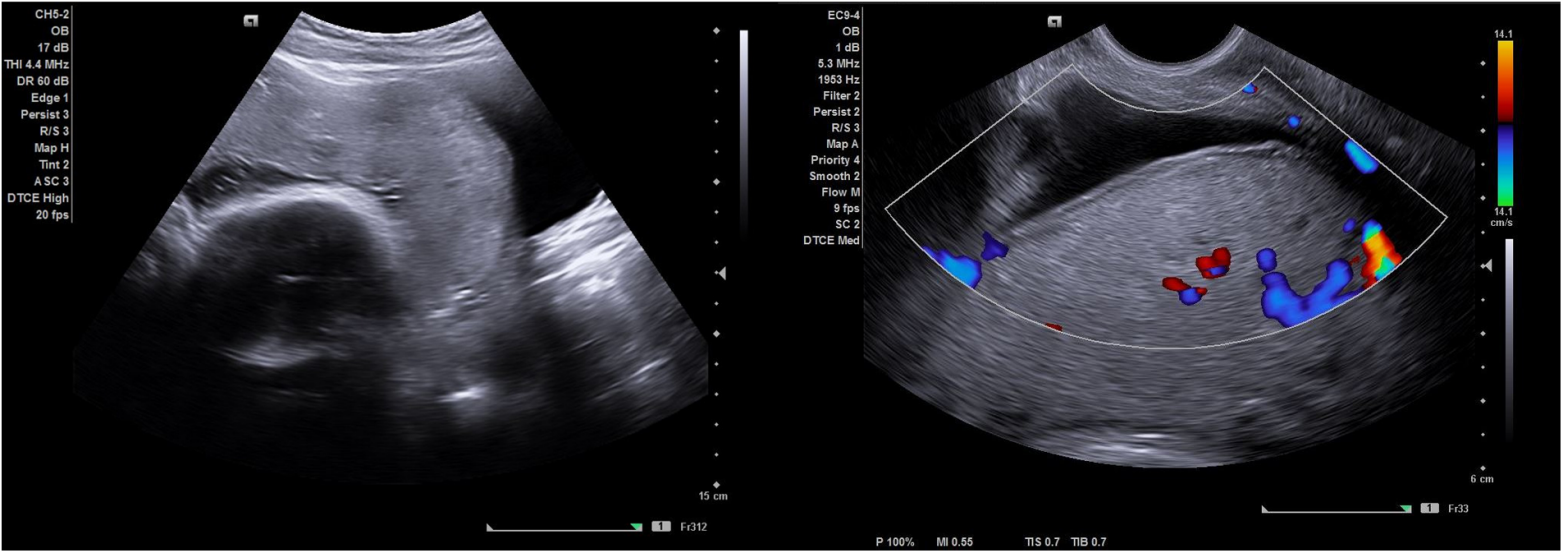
Shows an ultrasound image of a simple scar dehiscence overlying the placenta. Notice the absence of retroplacental hypervascularity, bridging vessels and abnormal lacunae (with no feeder vessels) observed on gray scale imaging and color Doppler.
